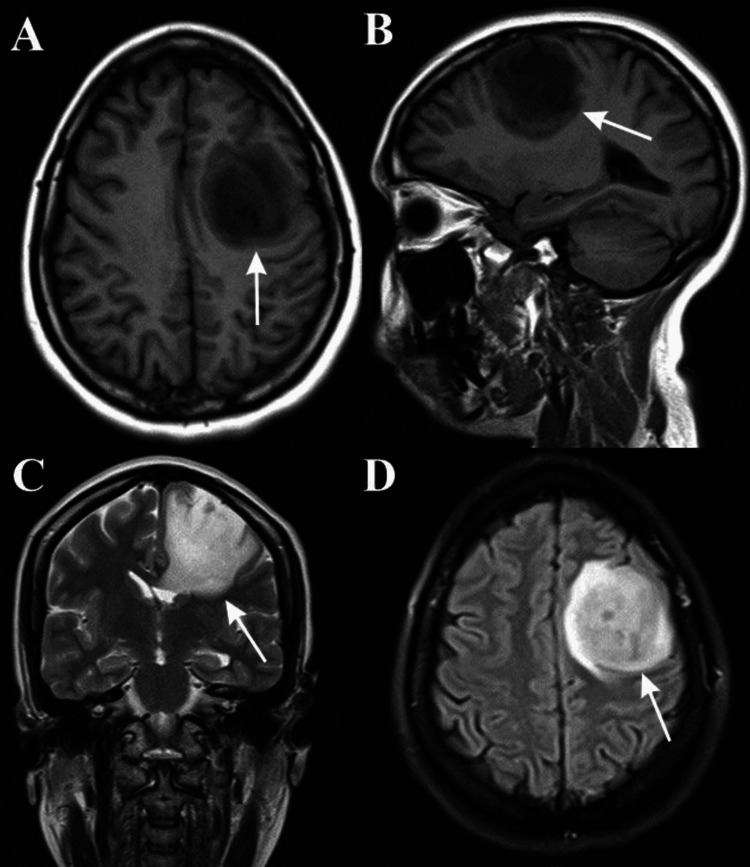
Initial brain magnetic resonance imaging Left frontal proliferative lesion of 46.9 x 43.6 x 52.6 mm causing deformation of the frontal gyrus and midline shift. The lesion was hypointense in the non-contrast axial (A) and sagittal (B) T1-weighted sequence, and hyperintense in the T2-weighted (C) and fluid-attenuated inversion recovery (FLAIR) (D) sequences.
